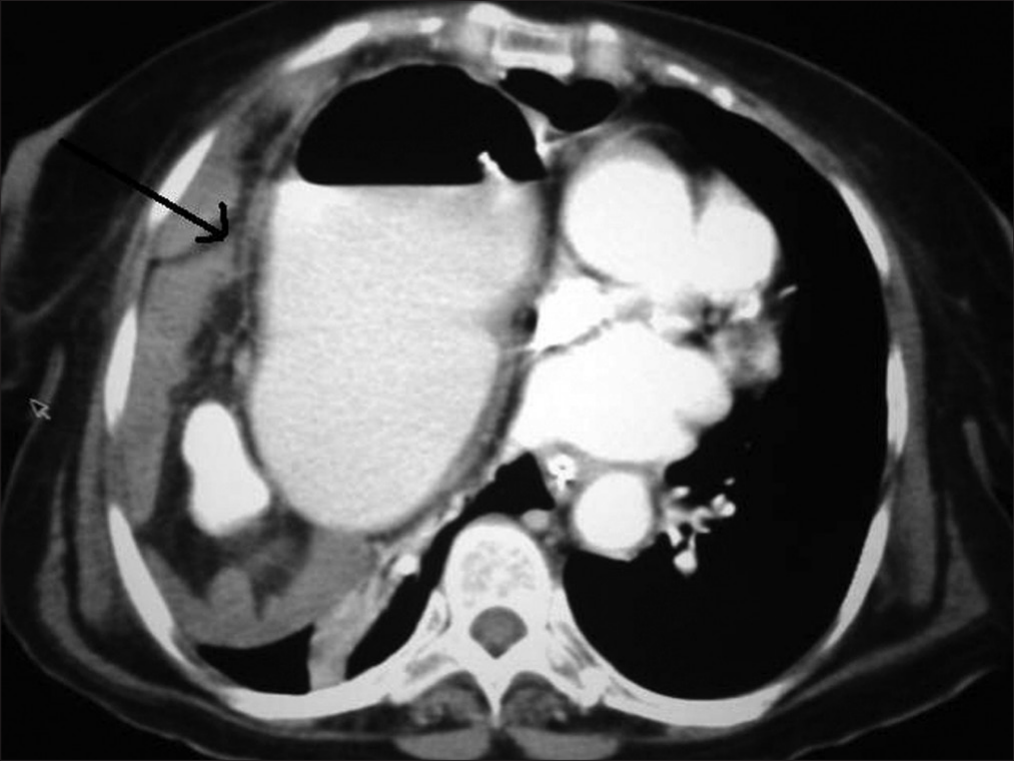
Computed tomography scan confirmed the diaphragmatic hernia with herniation of the stomach, pylorus, ascending and transverse colon into the right hemithorax (black arrow)
